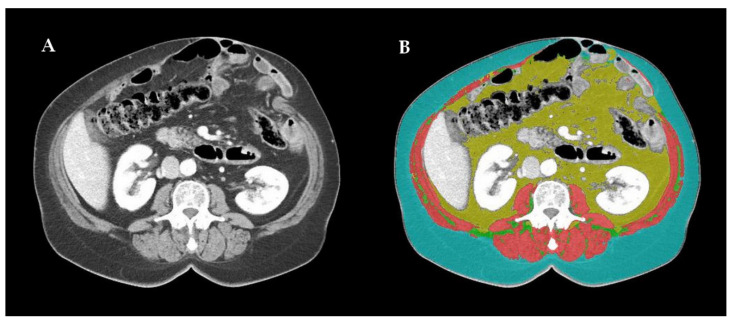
(A) Example of contrast-enhanced computed tomography scan of the third lumbar vertebra (L3) region in the venous phase (contains psoas, paraspinal and abdominal wall muscles); (B) image processed by Data Analysis Facilitation Suite ver. 2.0.5 by Voronoi Health Analytics, Vancouver, Canada with the Automatic Body composition Analyzer using Computed tomography image Segmentation module provided by Voronoi Health Analytics, Canada (2020); non-optimal segmentations were re-edited using open-source software 3D Slicer ver. 4.11 by 3D Slicer, Boston, MA, USA; skeletal muscles (pink) are separated from intra-abdominal fat (yellow) and subcutaneous fatty tissue (blue).
